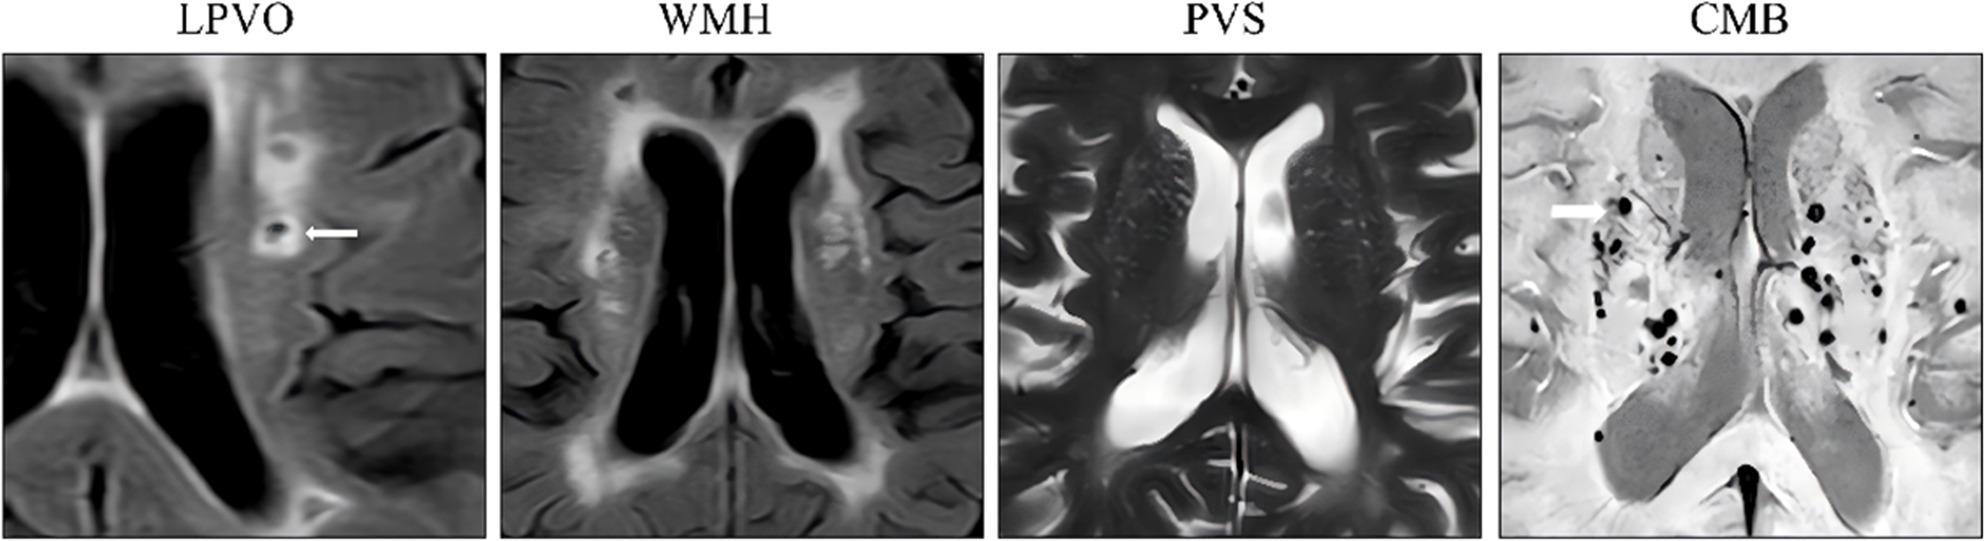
Representative MRI findings of CSVD lesions
